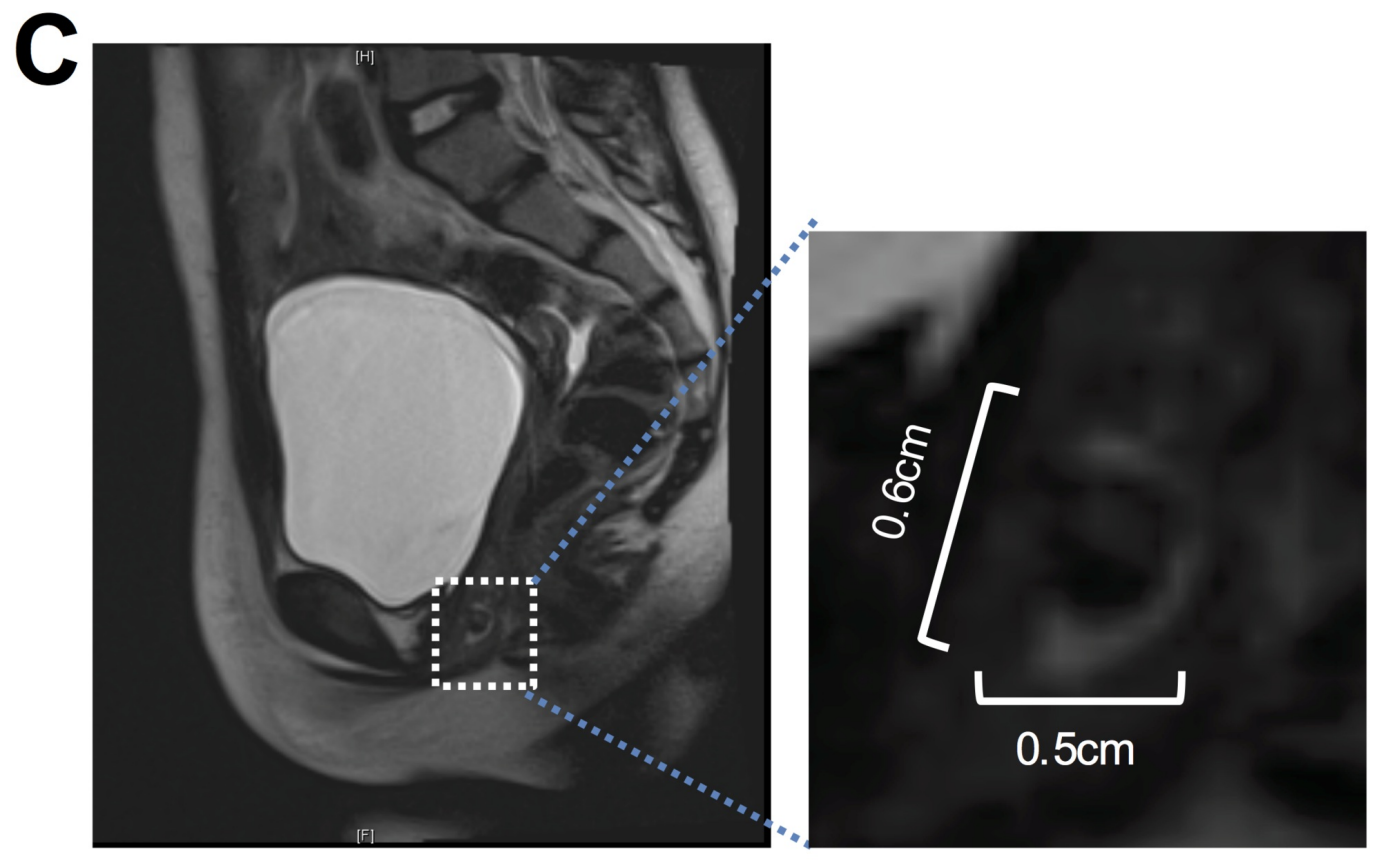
Contrast-enhanced MRI of the pelvis showing a non-enhancing filling defect within the vagina measuring 0.5 cm x 0.6 cm x 0.4 cm
